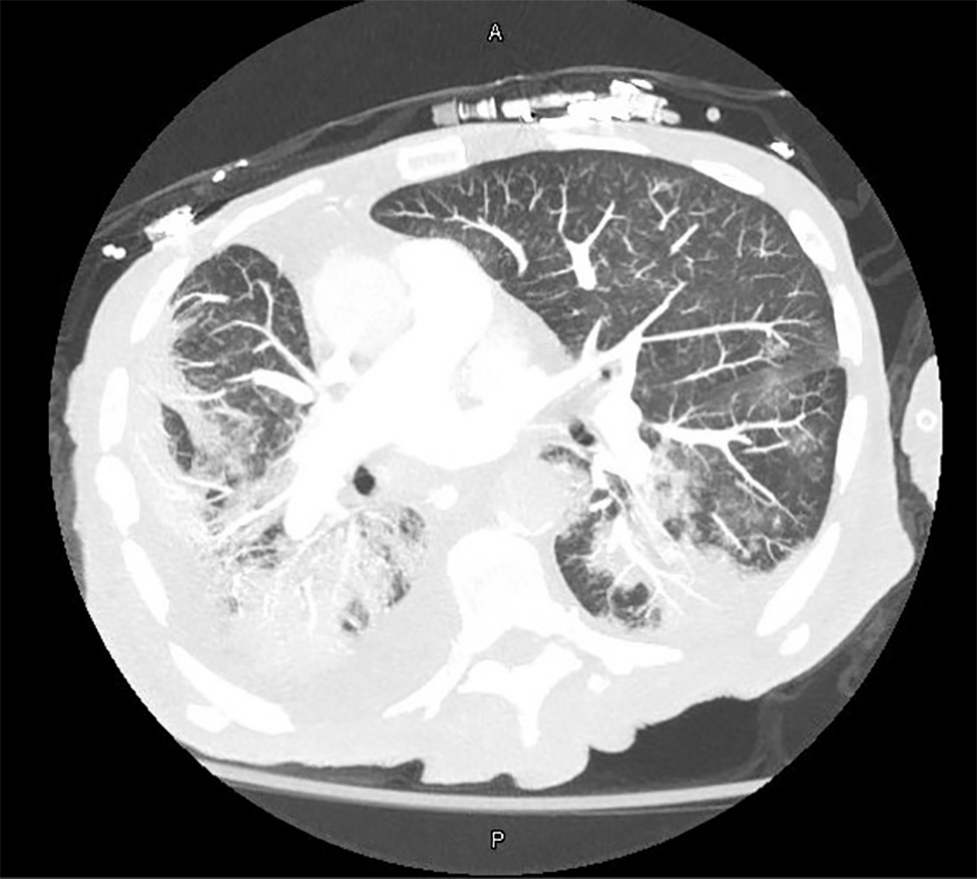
Computed tomography image of the chest showing bilateral dense consolidations and right-sided pleural effusion in 77-year-old man with severe acute respiratory syndrome coronavirus 2 who was later found to be co-infected with *Bordetella hinzii*. A, anterior; P, posterior
